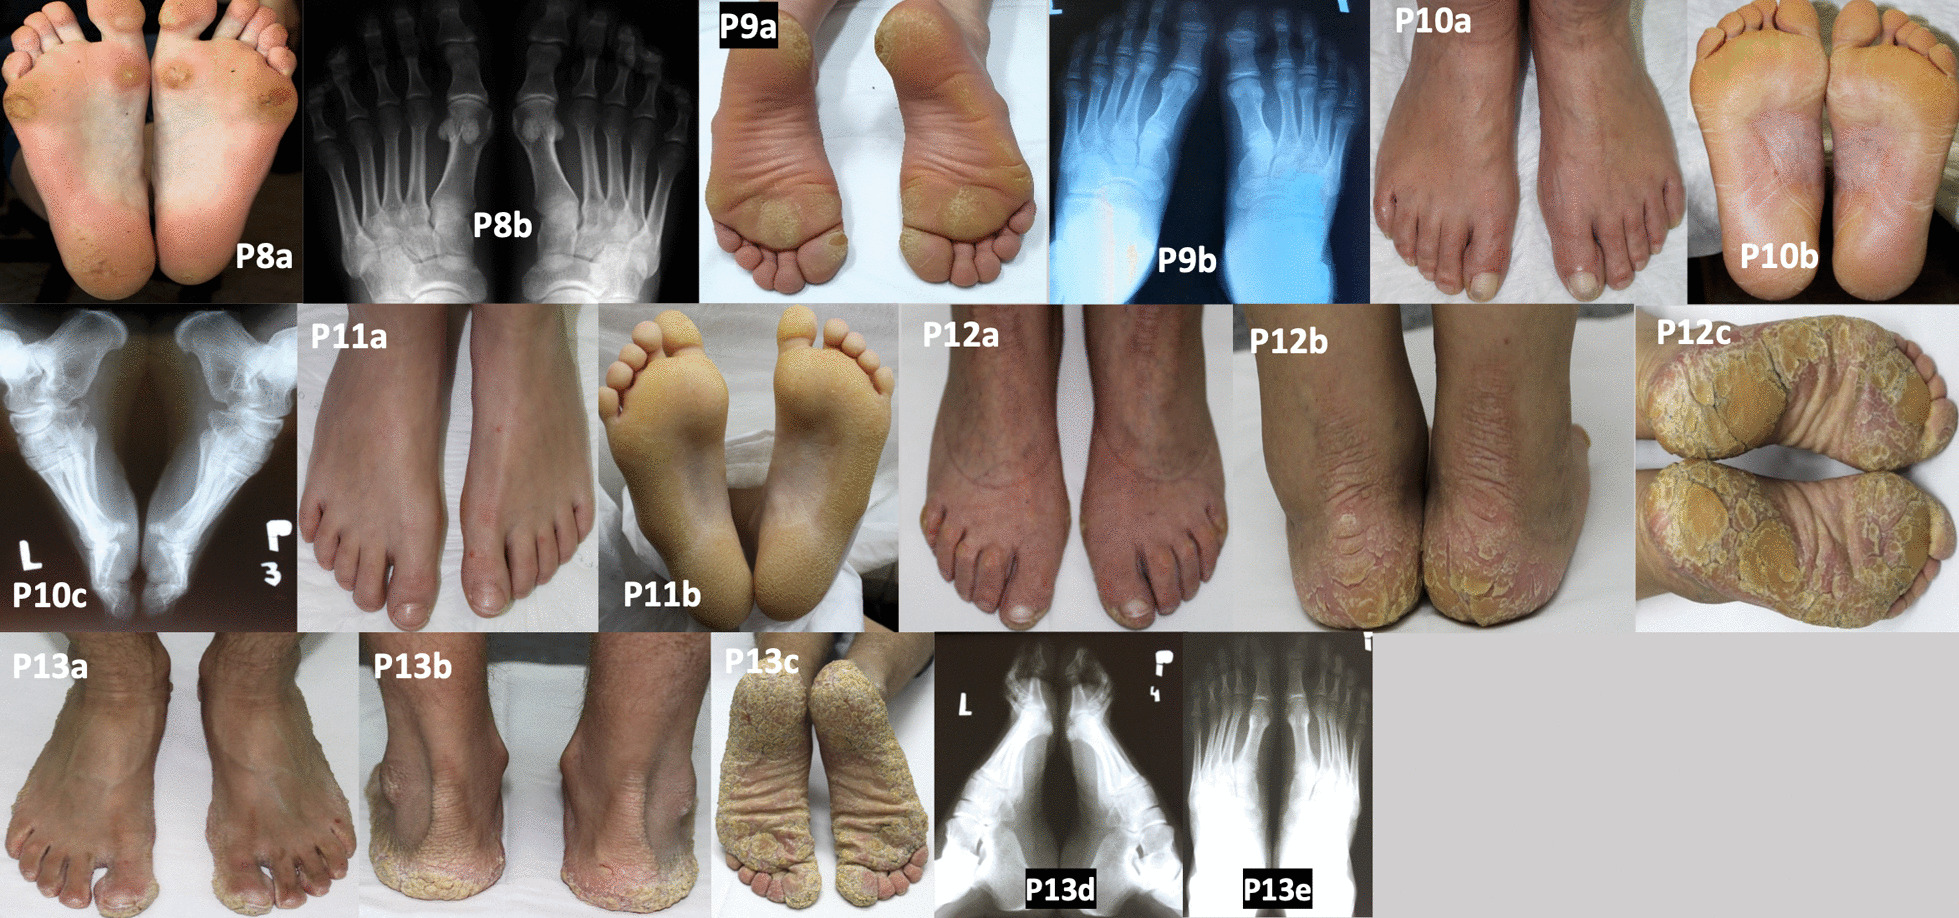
Patients with palmoplantar keratodermas. Areas of hyperkeratosis on the heels and foot arches and skin cracks on the right heel (P8a). Normal feet on a radiograph (P8b). Round areas of hyperkeratosis on the soles (P9a). Normal feet on a radiograph (P9b). Well-defined epidermal hyperkeratosis, numerous cracks in the epidermis arranged lenticularly, without fissures (P10a–b). Heel spur on a radiograph (P10c). Healthy skin on the dorsal feet (P11a). Well-defined enhanced epidermal hyperkeratosis and numerous cracks arranged reticularly, without fissures (P11b). Mild hallux valgity, claw toes II–V, with hyperkeratosis over interphalangeal joints II–V (P12a). Accentuation of the sulci over the Achilles tendon (P12b–c). Thick scaling on the skin of heels, soles, and metatarsal area, passing onto the forefoot in an arched manner, but not affecting the medial longitudinal arch of the foot (P12c). Healthy skin on the dorsal feet (P13a). Excessive epidermal furrowing and enhanced keratosis on the skin of the heels and over the Achilles tendon (P13b), with increased lesion severity on the skin of the heels, lateral surfaces of the foot, the entire forefoot, and the skin of the big toes (P13c). Normal foot radiographs (P13d–e)
